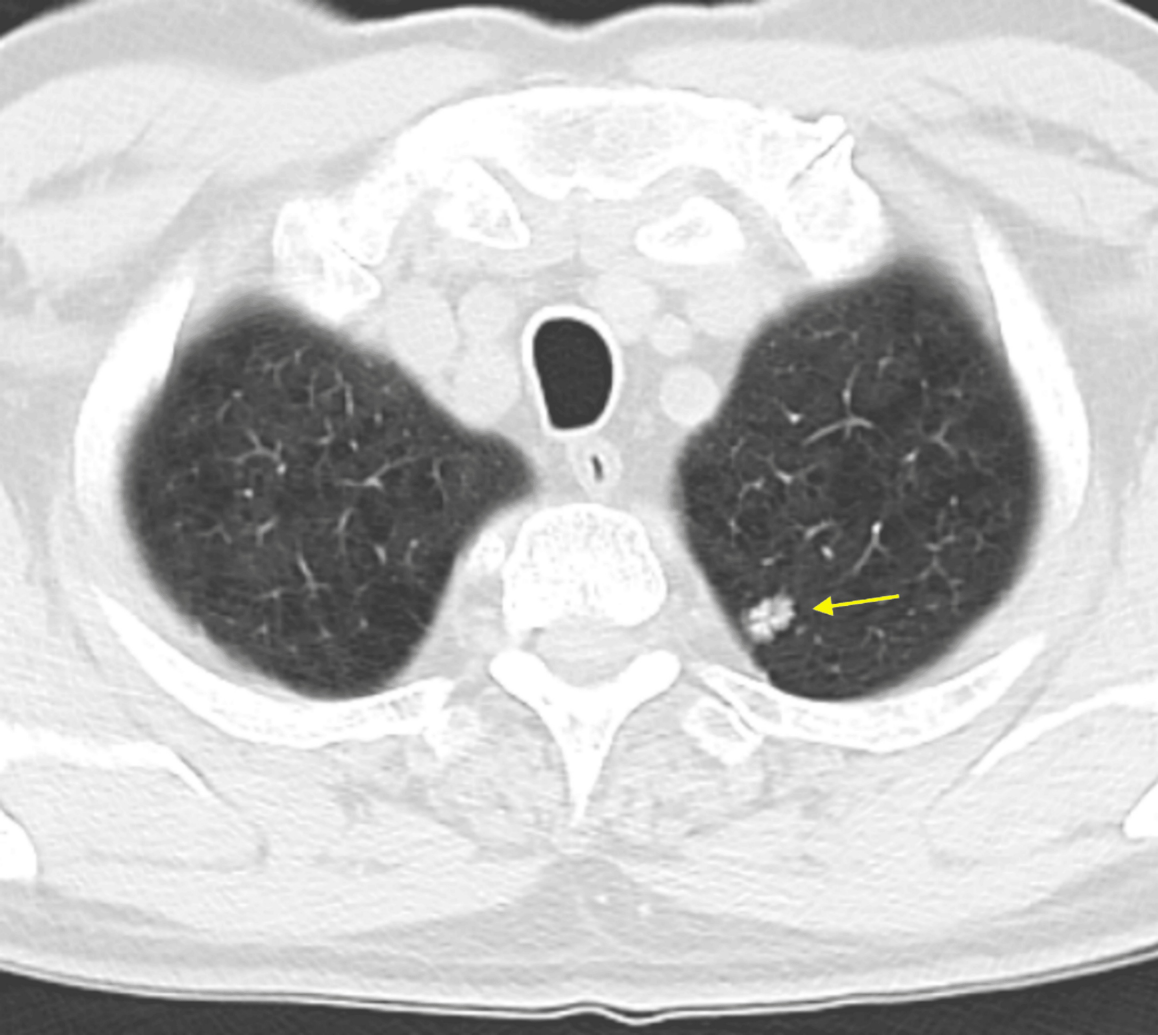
Chest CT scan in October 2024 showing a 11 x 8 mm nodule in the upper lobe of the left lung CT: computed tomography
